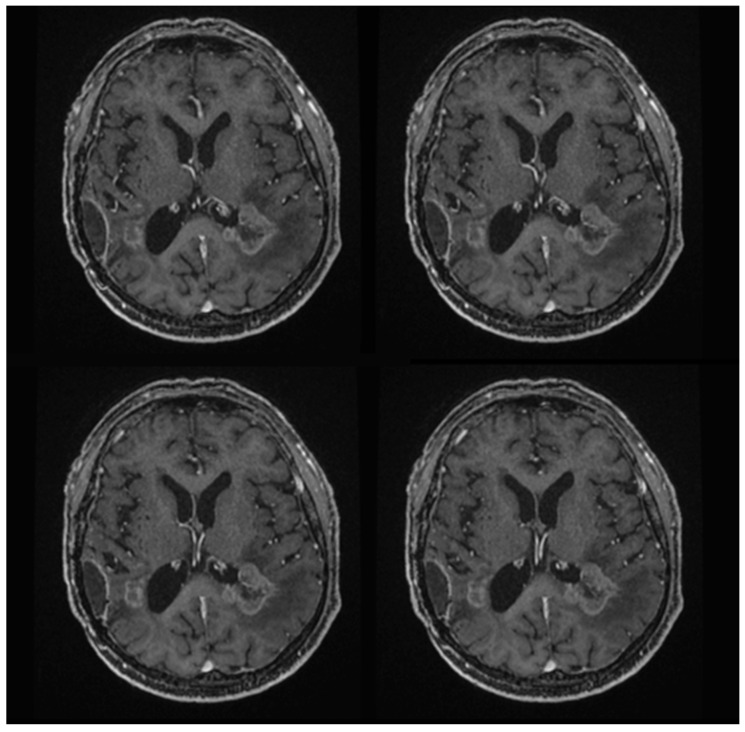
T1-weighted postoperative brain MRI sequences at more than one year of follow-up reveal two new brain lesions: one in the right para-trigonal area and another infiltrating the splenium of the corpus callosum on the left side. There is no evidence of recurrence of the previous lesions on the right.
